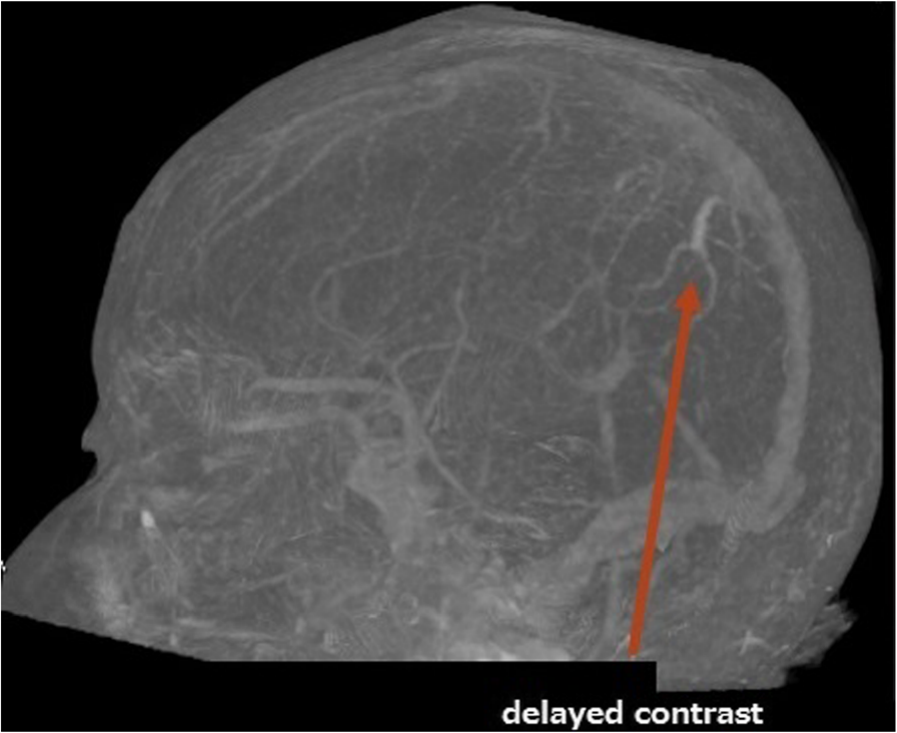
A time delay computed tomography-digital subtraction angiography image obtained at admission showing the culprit cortical vein. This vein coincided with the occluded cortical vein shown in the intraoperative image (Fig. 2)
